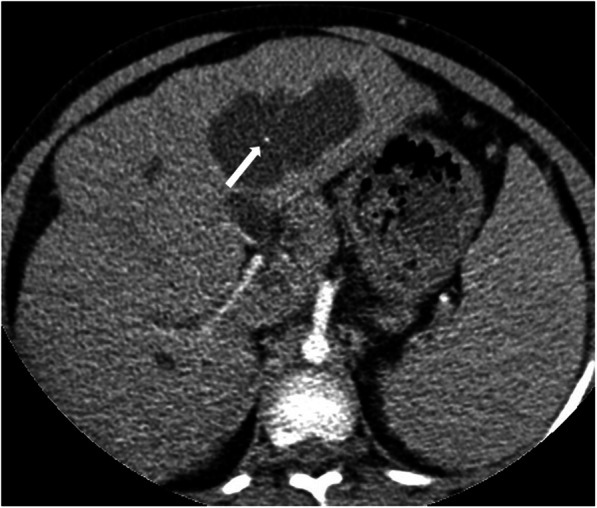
A 6-year-old male patient with known Caroli syndrome. Axial plane arterial phase CT image shows central dot sign (arrow)
